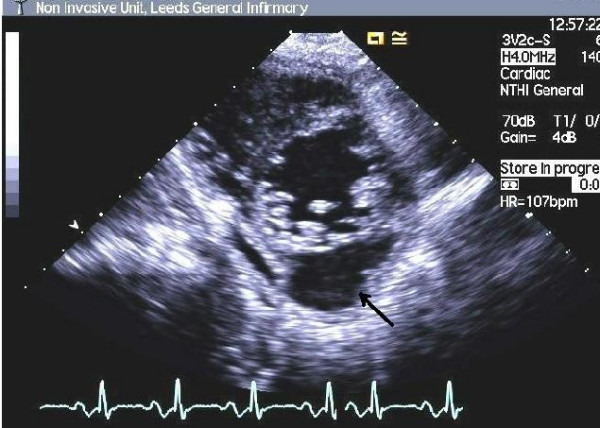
Transthoracic echo – Short axis view showing abscess cavity.
